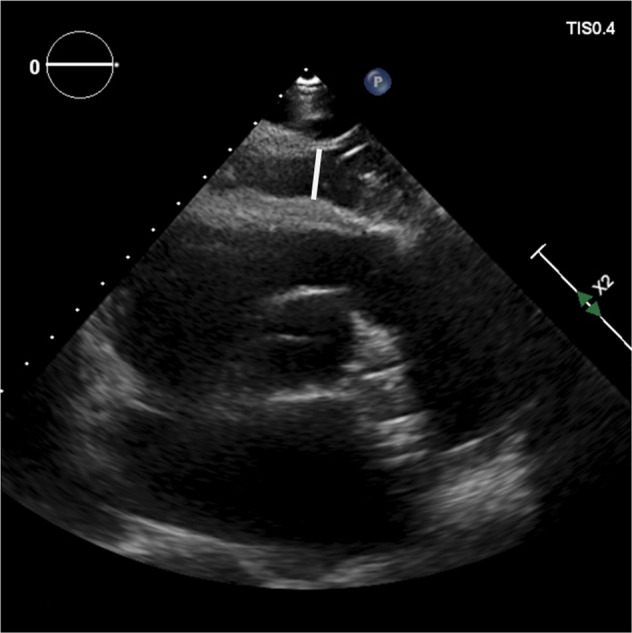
Example image of a EAT measurement in parasternal short-axis view. The EAT thickness is measured as space between the epicardial wall of the myocardium and the visceral layer of the pericardium.
